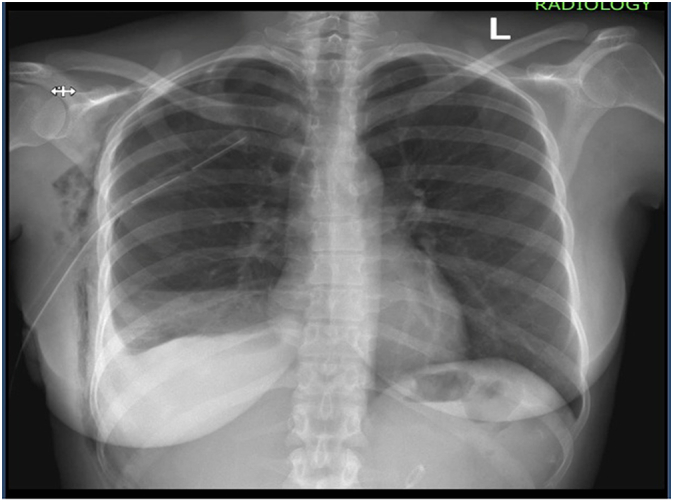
Control X-ray following chemical pleurodesis revealing mild right-sided effusion.
